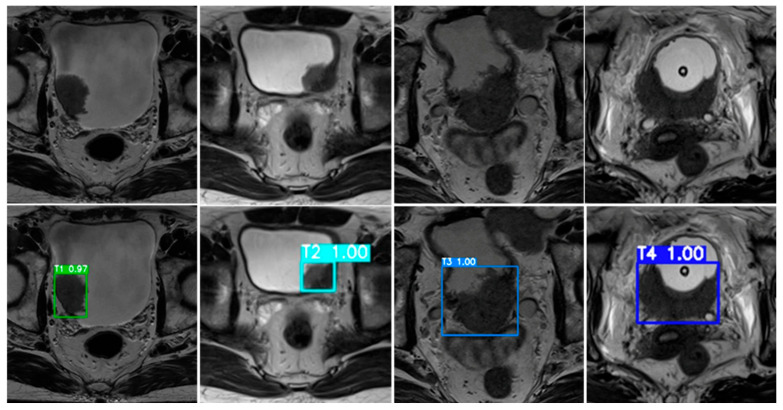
Representative MRI-based bladder cancer stage predictions generated by the proposed AI framework using the ResNet50 model—the best-performing classifier in this study.
